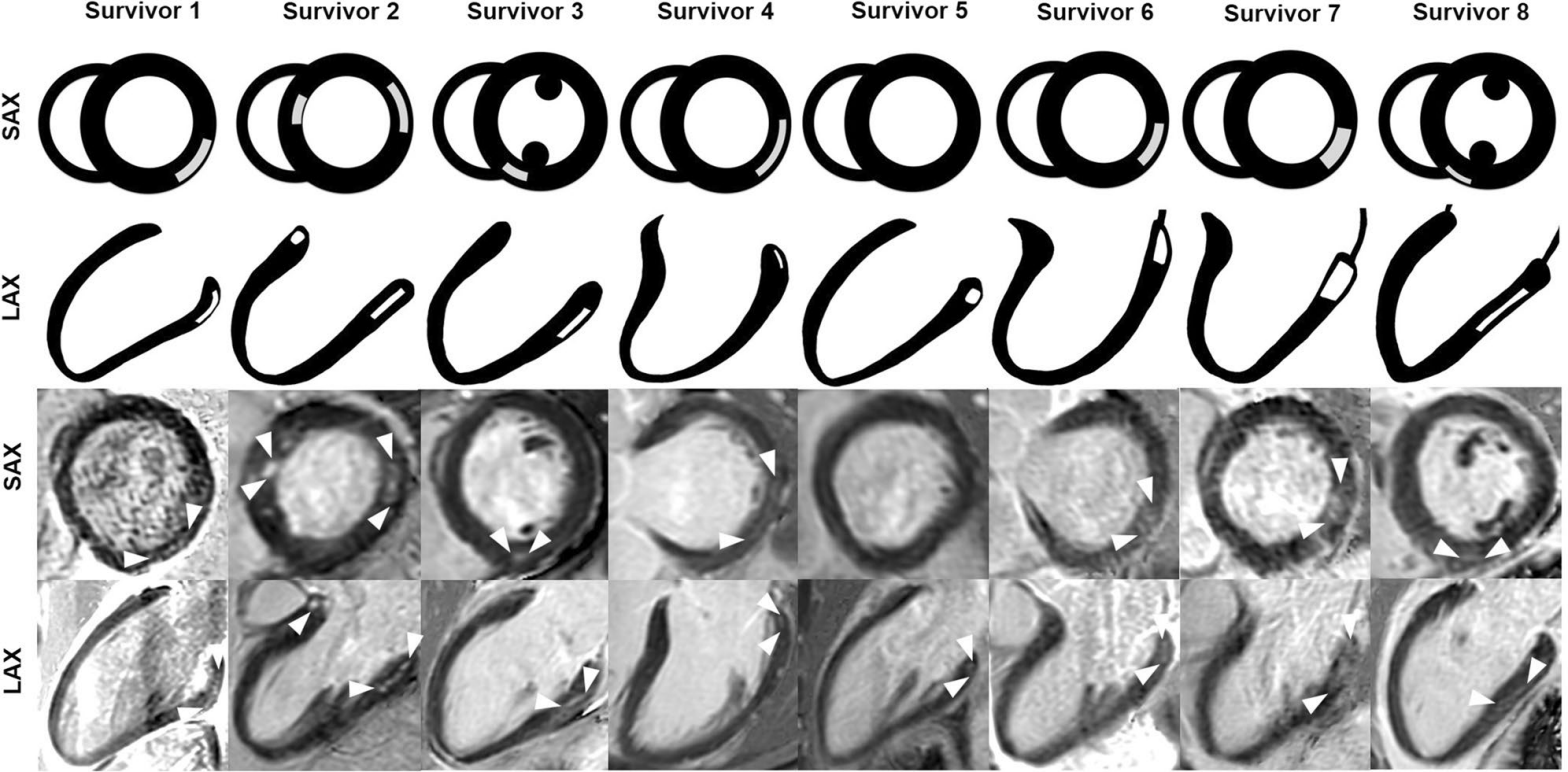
Non-ischemic LGE in germ cell cancer survivors. Myocardial tissue was characterized by cardiac magnetic resonance imaging in asymptomatic germ cell cancer survivors after platinum-based chemotherapy (*n* = 44) and age-matched healthy controls (*n* = 21) by late gadolinium enhancement (LGE) imaging. Short-axis and long-axis images of eight germ cell cancer survivors with non-ischemic LGE are shown. Arrows indicate non-ischemic LGE lesions. LGE lesions are predominantly located at subepicardial and mid-wall left ventricular segments. Two patients had LGE lesions located at the posterior right ventricular insertion point (survivors 3 and 8)
